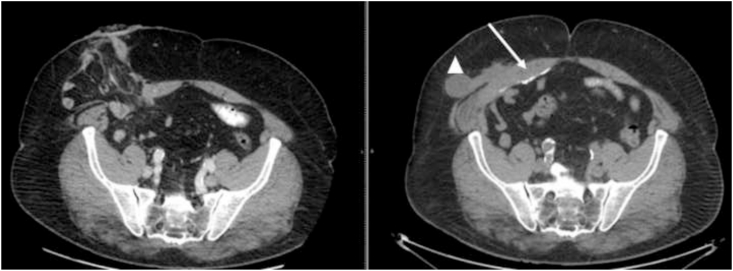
A comparison between the pre-operative CT-scan and that performed after 5 months from surgery: the row indicate the complete repair of the hernia. A triangle indicate a small seroma about 2 cm that is now reabsorbed.
